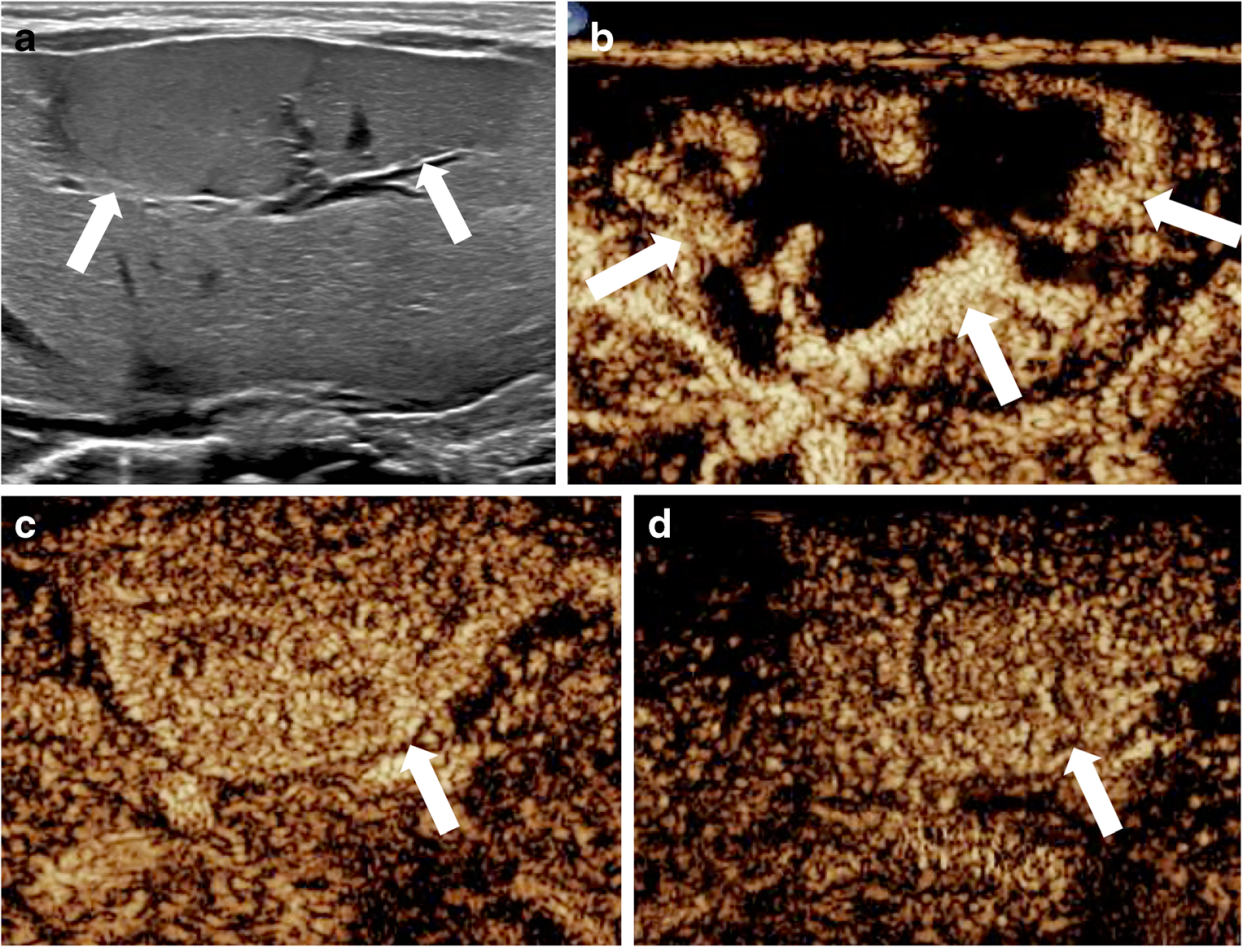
Congenital hemangioma in a 2-week-old boy with elevated liver enzymes. a Transverse gray-scale US demonstrates a large focal liver lesion (*arrows*). b–d Transverse contrast-enhanced ultrasound (CEUS) images in contrast-only mode during dynamic enhancement phases. In the arterial phase (b), the lesion demonstrates peripheral, nodular, discontinuous enhancement pattern (*arrows*). In the portal venous phase (c), the lesion (*arrow*) appears homogeneously hyperenhancing. In the late phase (d), the lesion (*arrow*) remains hyperenhancing compared to the adjacent liver parenchyma. See Online Supplementary Material 1 for cinematic clip
